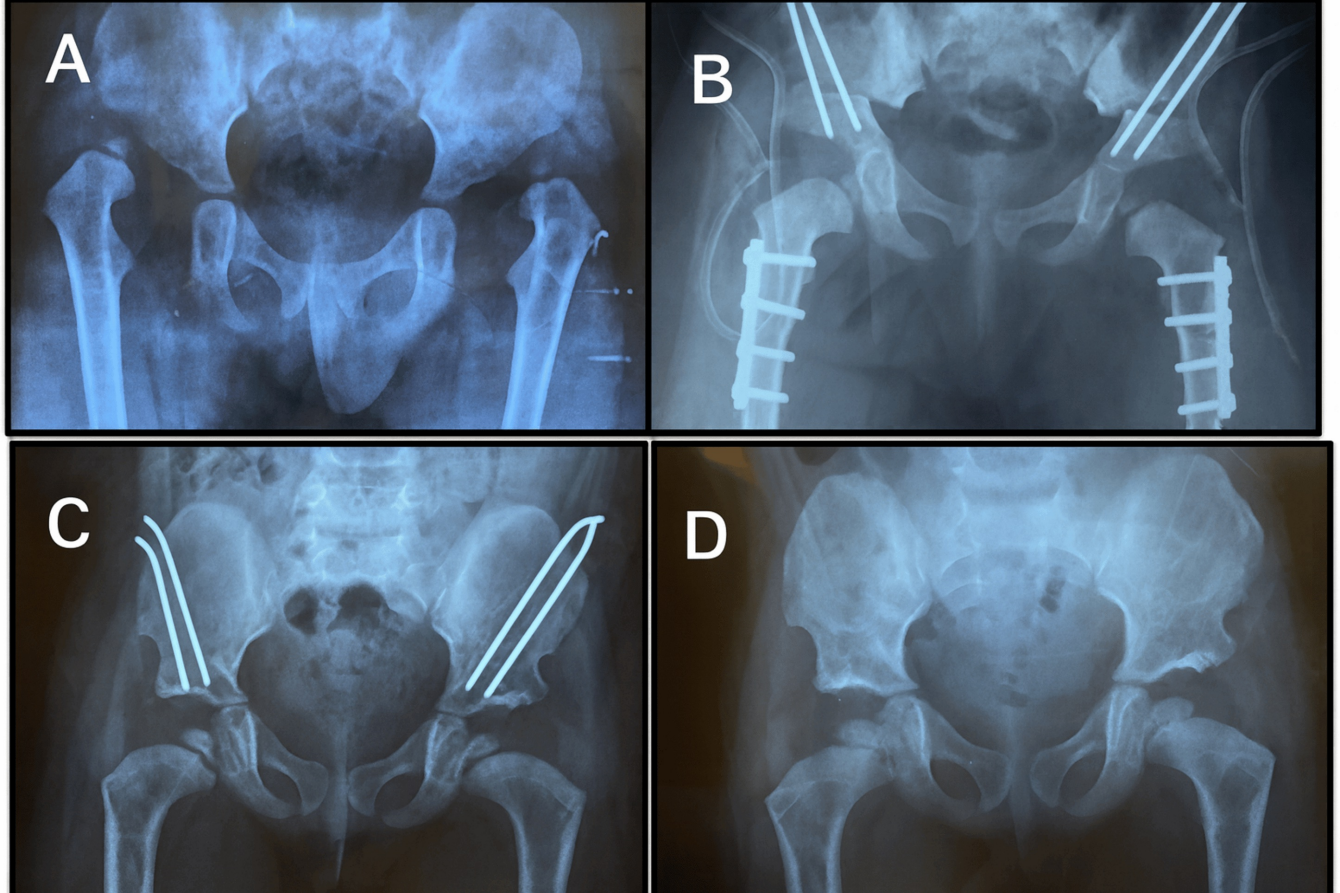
Radiographic progression in a 41-month-old female patient: (A) preoperative appearance, (B) immediate postoperative result, (C) follow-up at 10 months post-operation, and (D) follow-up at 14 months post-operation
